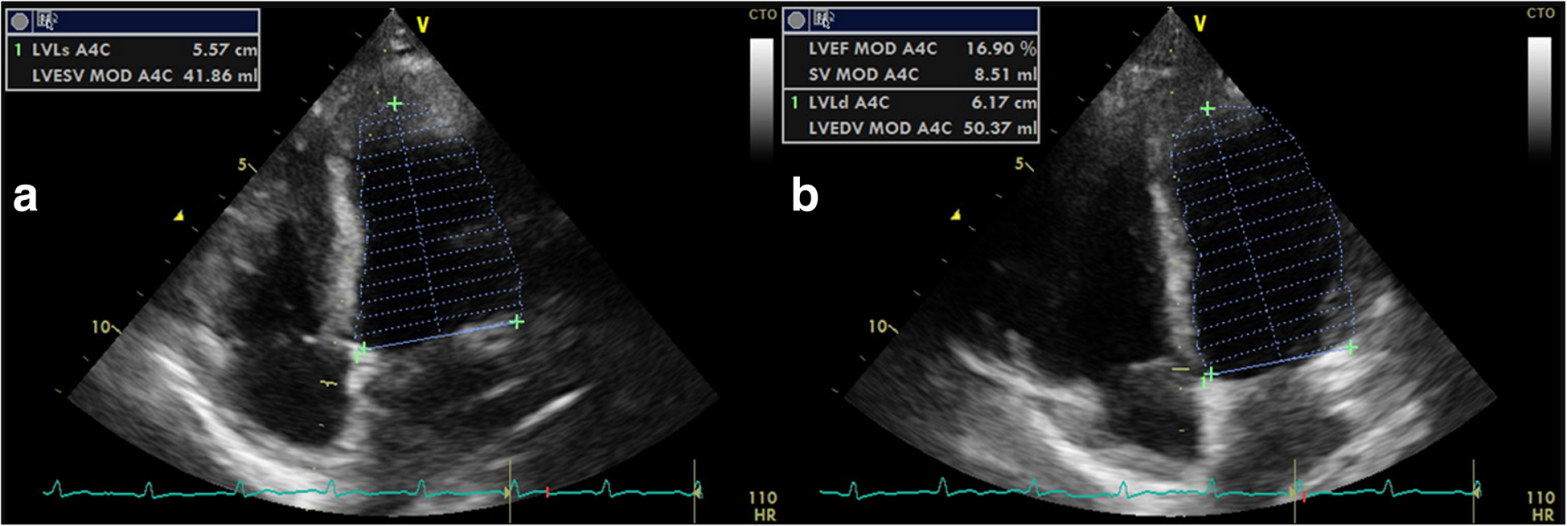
Echocardiographic findings via apical four chamber views showing (a) end-systolic and (b) end-diastolic volumes. The echocardiography showed a dilated, spherical ventricle with reduced systolic function (left ventricle ejection fraction, 17%) and pericardial effusion
